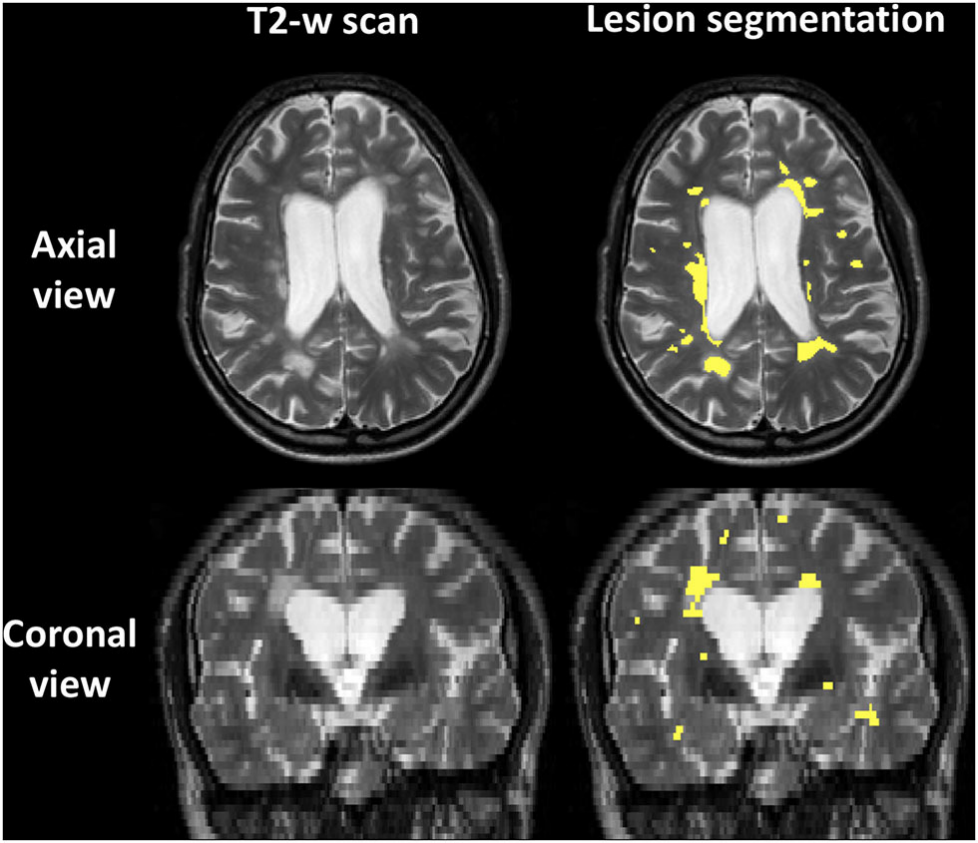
Example of lesion segmentation on the T2-weighted fast spin echo 2D anatomical scan of one patient. Top: axial views; bottom: coronal views. Left: scan; right: scan with lesions outlined in yellow.
